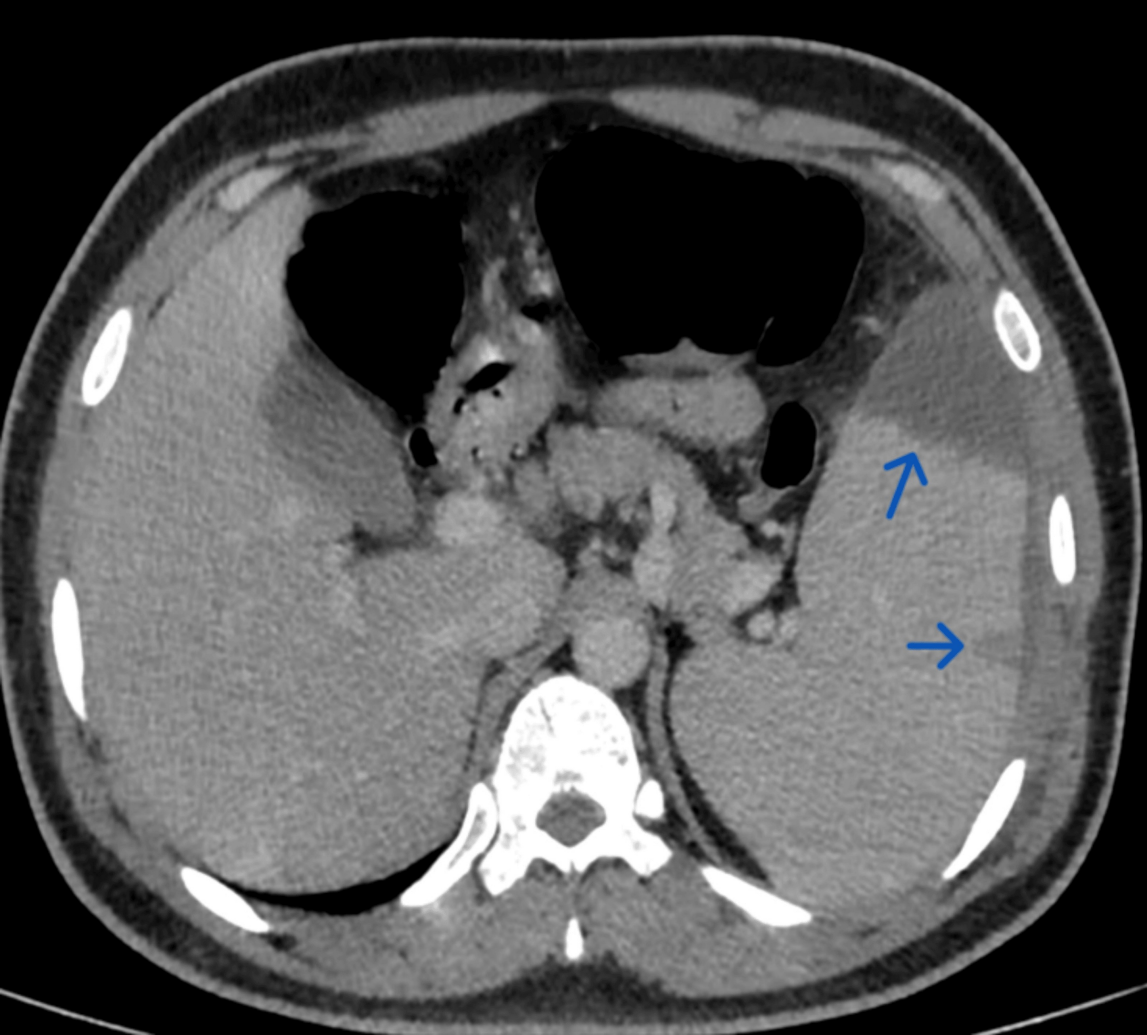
Contrast-enhanced computed tomography scan of the abdomen, axial section shows multiple wedge-shaped hypodense non-enhancing areas, suggestive of splenic infarcts (blue arrows)
